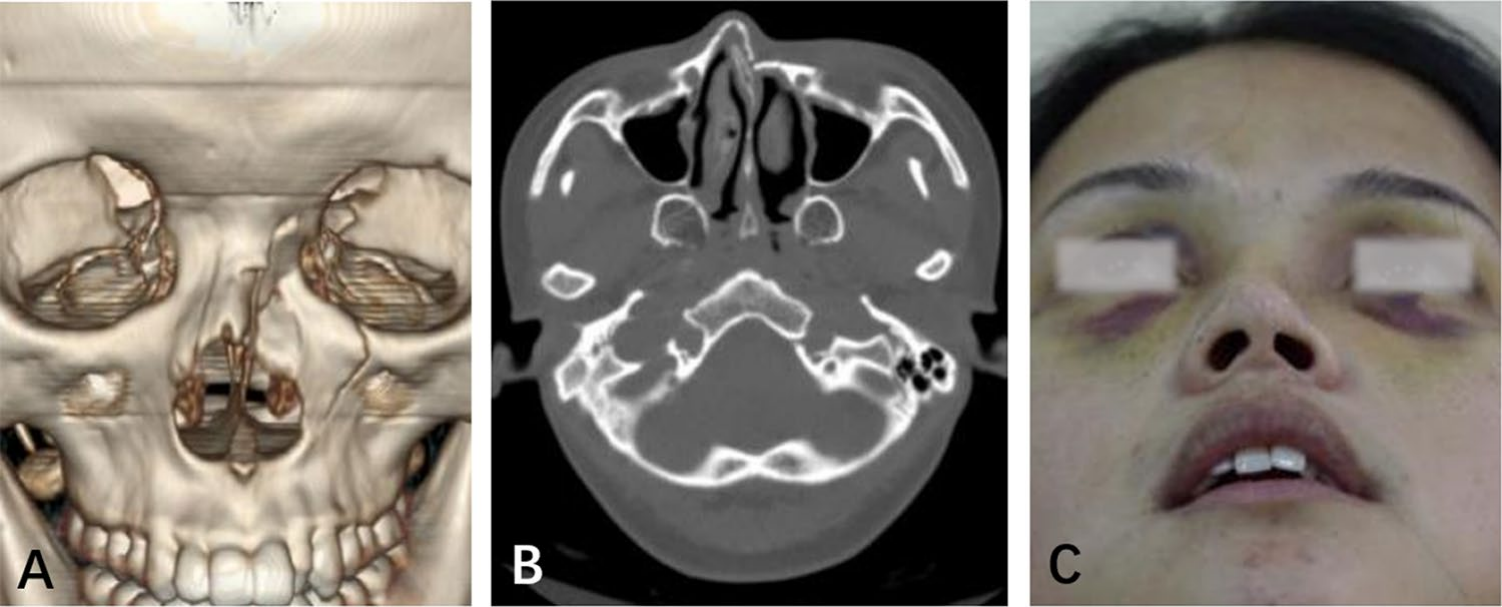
Type I fracture in the left pyriform buttress area. Three-dimensional and horizontal computed tomography images reveal the collapse of the left upper third of the nasal vault (A, B). A slightly asymmetry of the nostrils was observed from the head up view (C)
